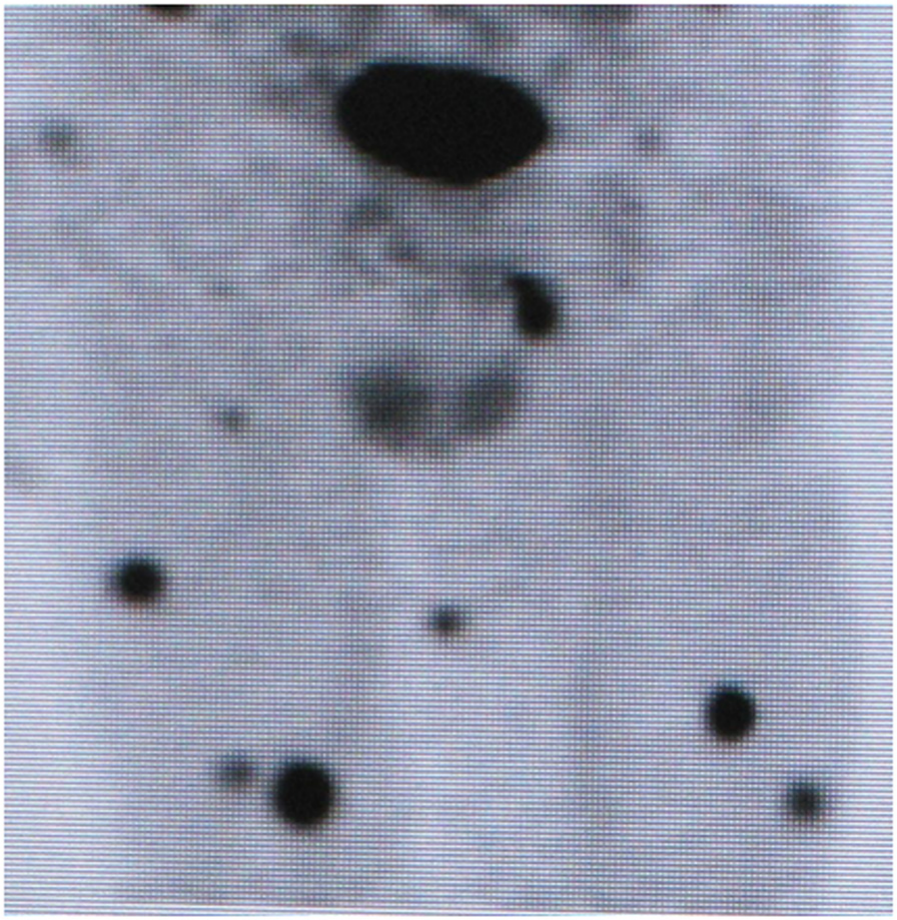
Positron emission tomography-computed tomography scan. Positron emission tomography-computed tomography scan shows multiple skeletal muscle metastases in the bilateral thighs.
